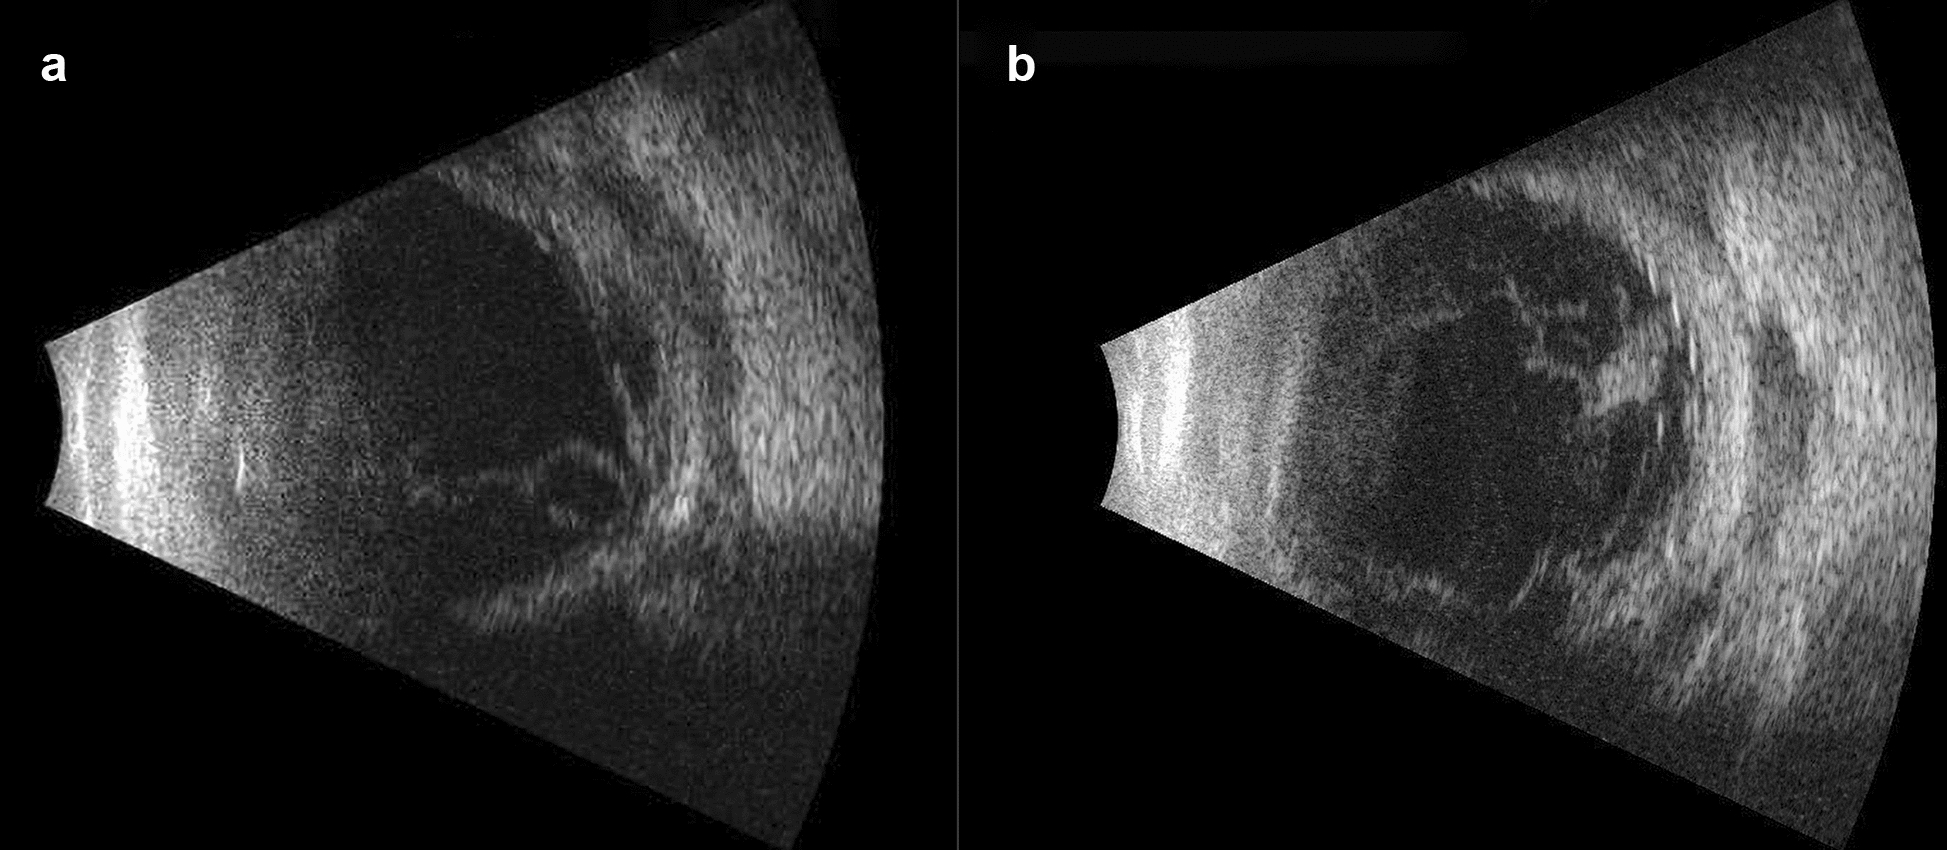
A B-scan Ultrasonography of left eye showing low reflectivity and thickening of the sclera at wound site. B B-scan Ultrasonography of left eye demonstrating more vitreous opacities with retina and choroid (RC) layer thickening suggesting endophthalmitis
